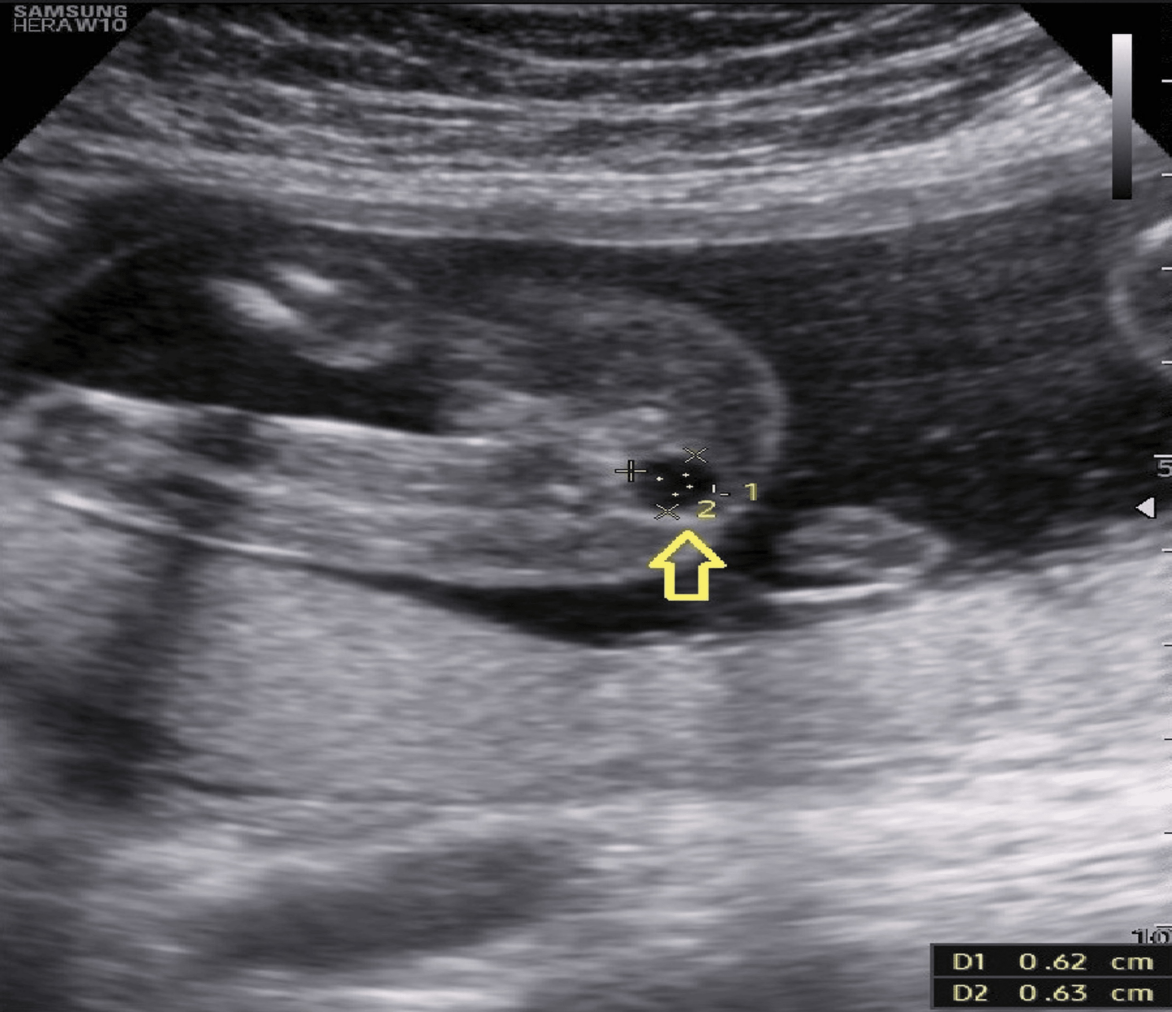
Ultrasound (transverse plane) at 21 weeks of the affected fetus showing a presacral cystic mass (arrow)
